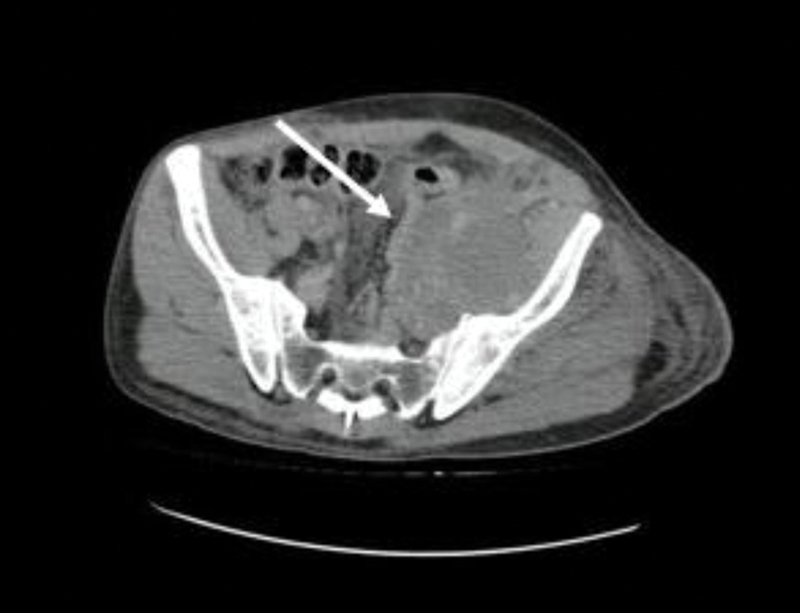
Axial view of an abdominal CT, with a soft tissue mass infiltrating the left psoas muscle (arrow).
